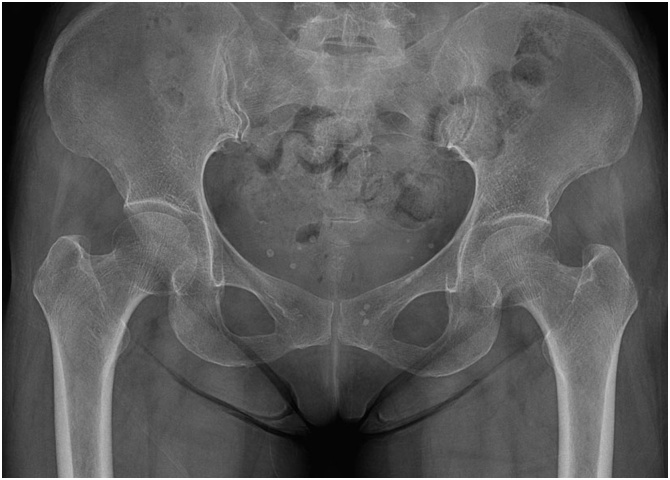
Radiographs of patient’s pelvis showed no evidence of fractures on Lt. hip.
